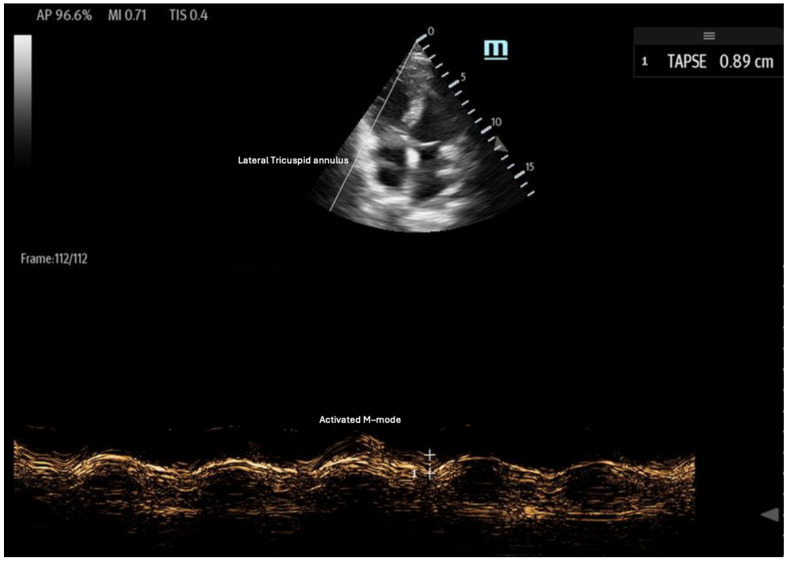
Tricuspid annular plane systolic excursion (TAPSE): apical 4-chamber view with M-mode activation, demonstrating reduced TAPSE (measured at the bottom 2D M-mode).
